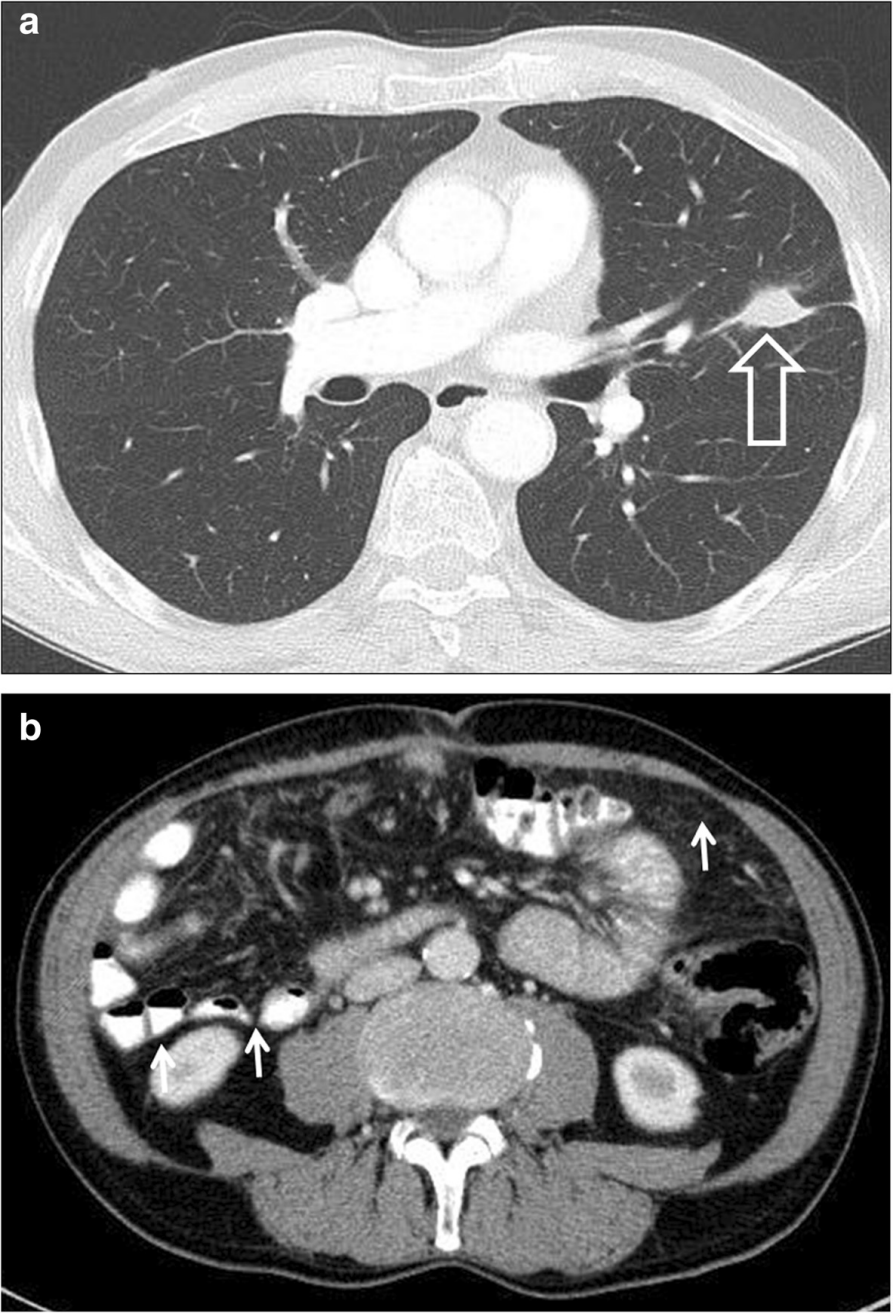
Contrast-enhanced computed tomography scan of the chest and abdomen after treatment with catumaxomab in July 2012. (a) Partial response of the pulmonary lesion (1.8 × 1.1 cm, big arrow) and (b) regression of peritoneal carcinomatosis (small arrows) with less amount of ascites.
